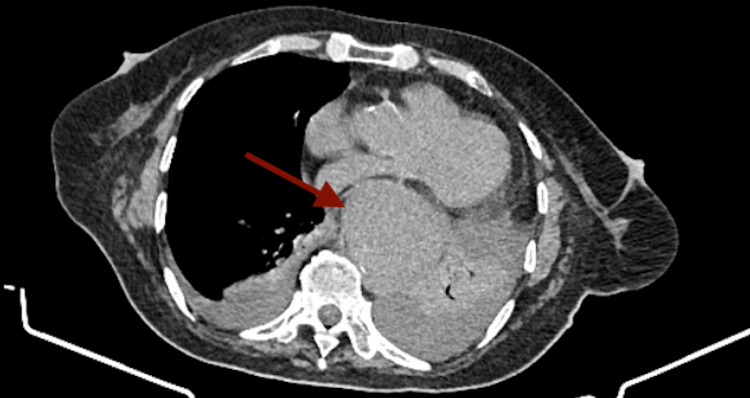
Computed tomography depiction of aortic intramural hematoma (arrow) increasing the diameter of thoracic aorta to 6.5 cm.
